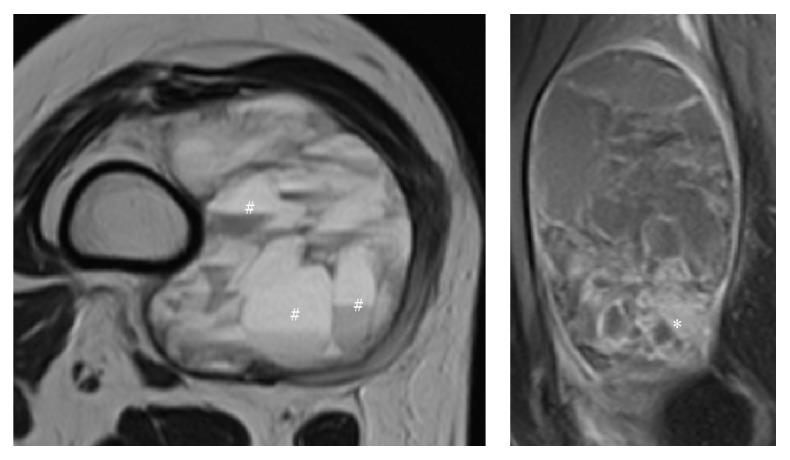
MRI characteristics of soft tissue sarcoma with telangiectatic changes (STST) images showed typical MRI features of STST: (#) fluid-fluid levels, (*∗*) tumor nodules.
